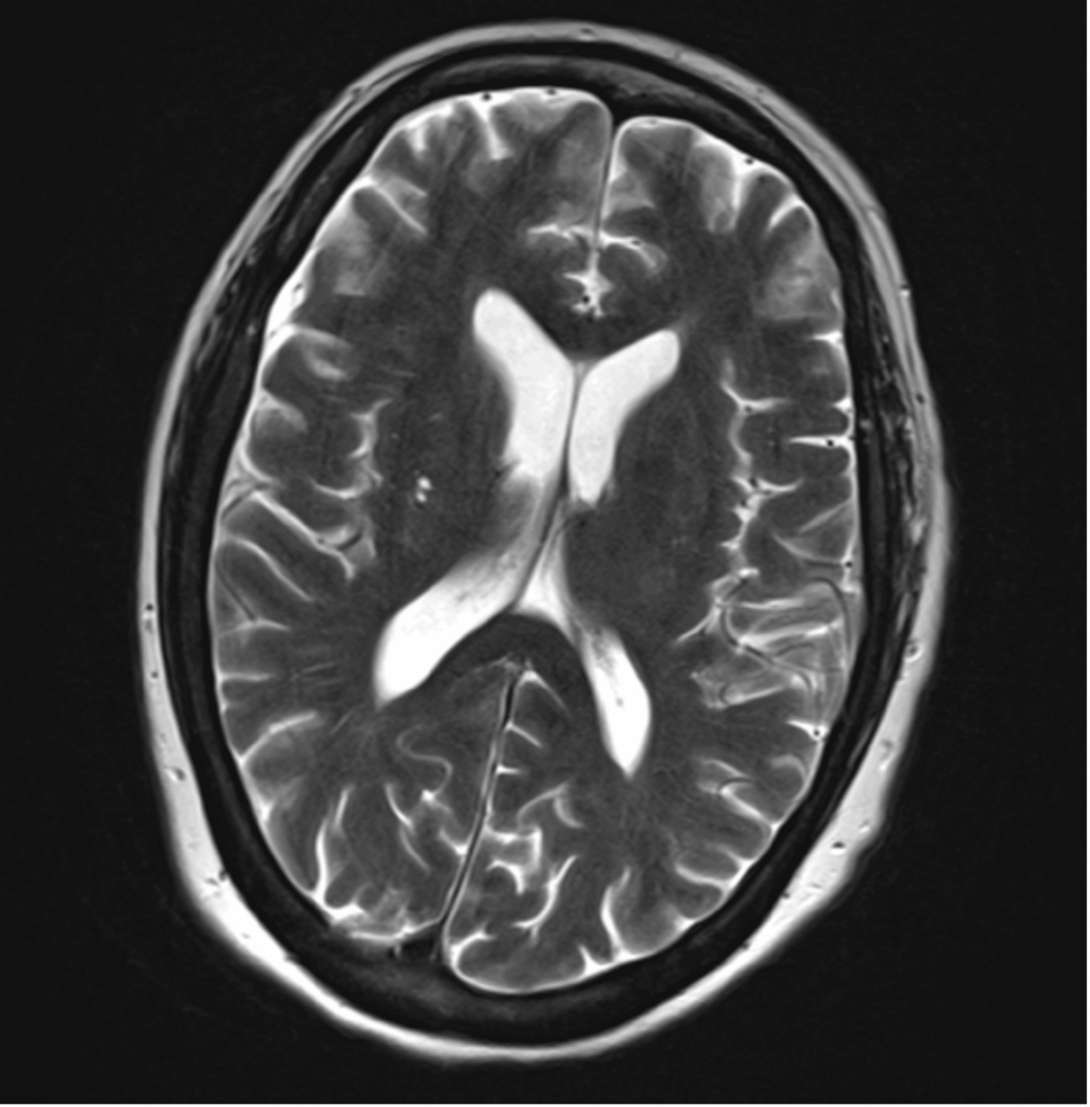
Brain MRI of the patient one day after admission MRI: magnetic resonance imaging
